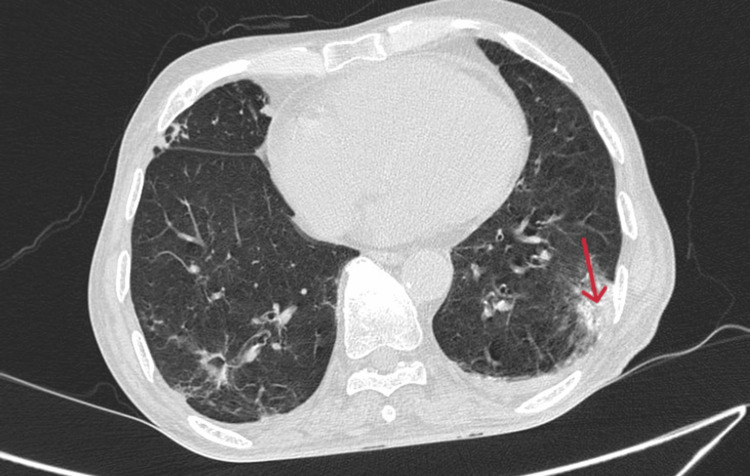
Axial CT lung window showing pulmonary infarct in the left lower lobe. CT, computed tomography
